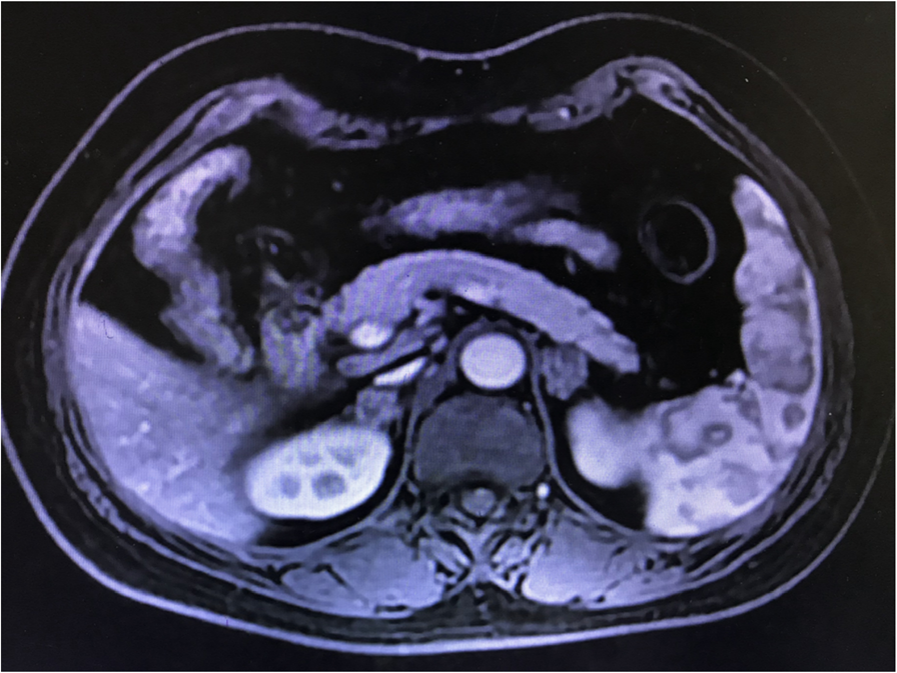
Enhanced magnetic resonance imaging (postoperative day 140) revealed a clearly shrunken adrenal mass compared with 3 months ago
